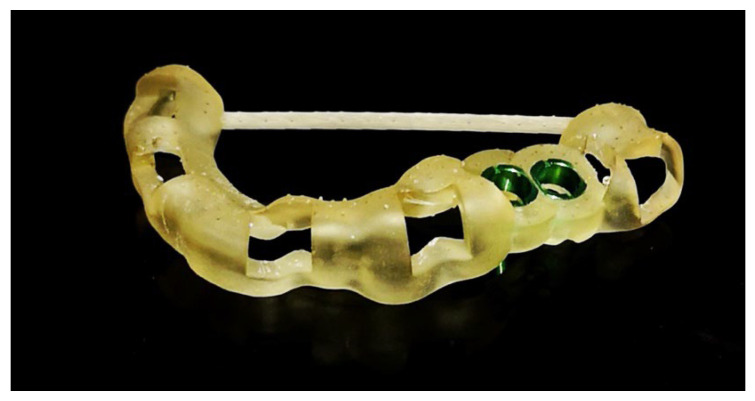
Surgical templates printed using a 3D printer personalized to the patient’s anatomical features, fitted on CT scan and oral scanner. Used to facilitate and speed up the surgical procedure of dental implants. Material from the authors’ collection.
